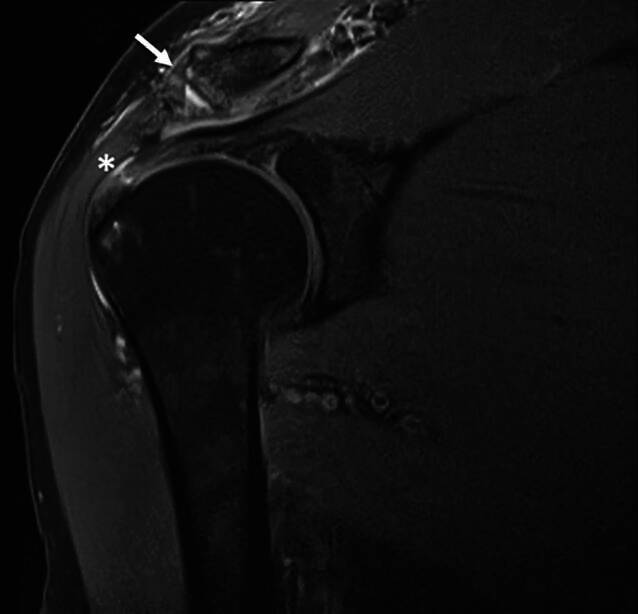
DW-MRI of the right shoulder showing effusion around the degenerative changed AC joint (arrow), asterisk: transmural lesion of supraspinatus tendon
